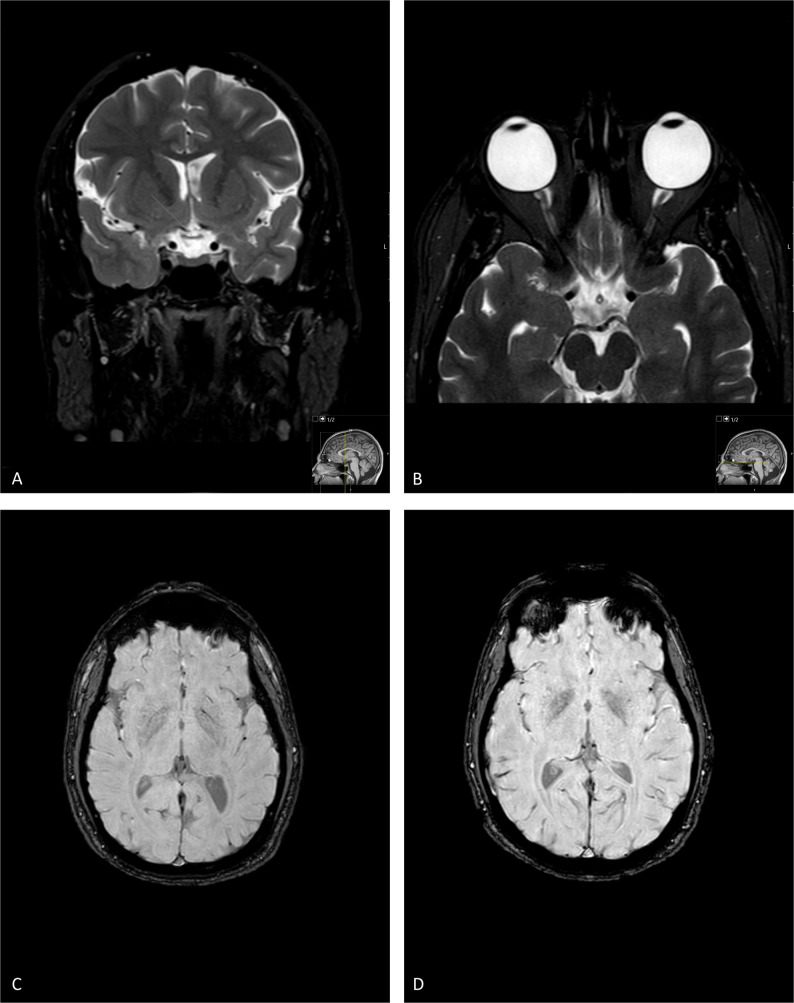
Brain MRI. T2 coronal (A) and axial (B) scans of patient 1 demonstrate thinning of the optic nerves and chiasm, with no other pathological findings. Susceptibility-weighted imaging (SWI) of Patient 1 (C) and Patient 2 (D) revealed no abnormal iron accumulation within the basal ganglia
